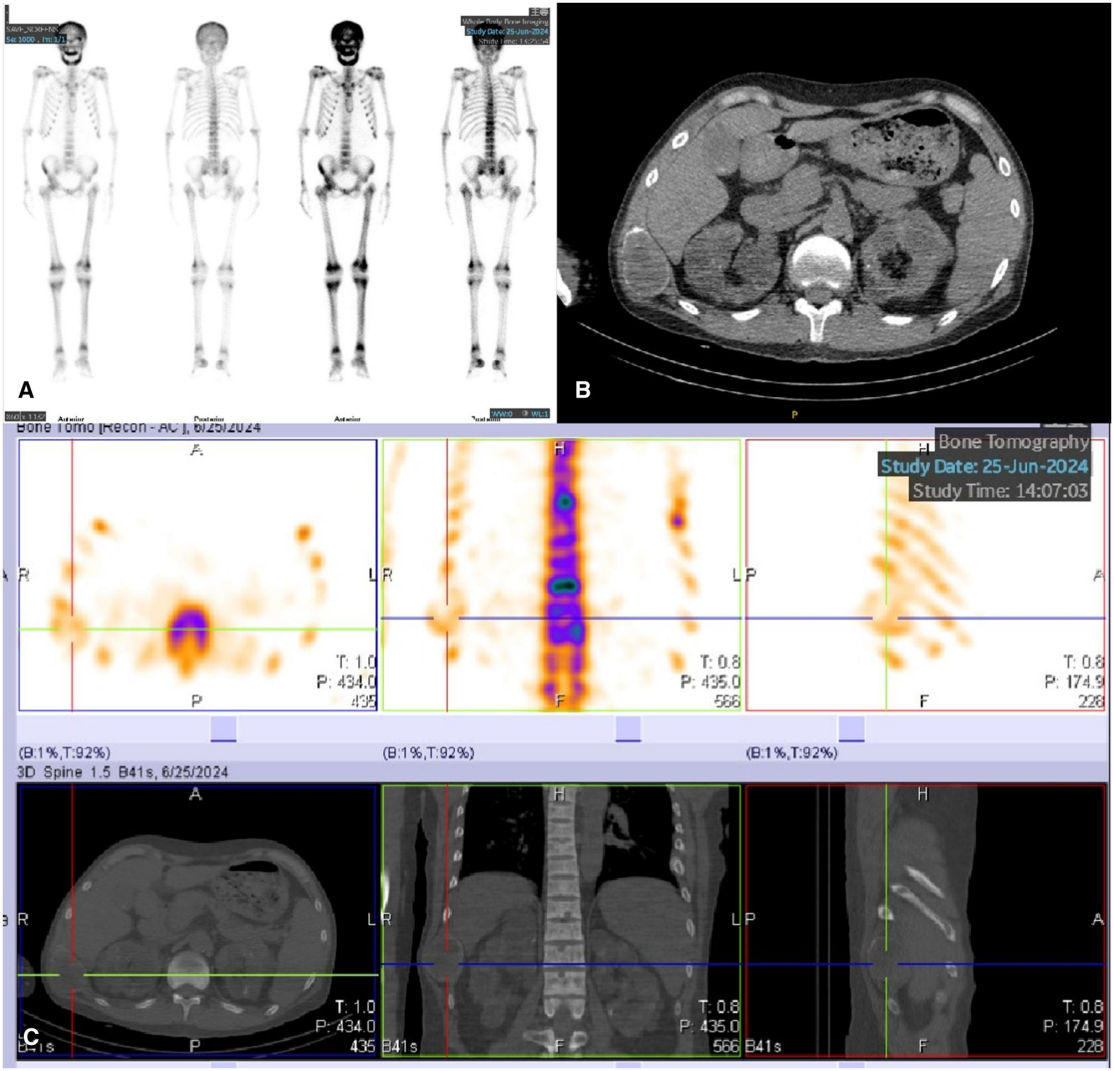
(A) The whole-body bone scan demonstrates diffuse tracer uptake in the skull, with abnormally increased radiotracer accumulation observable in the clavicles, ribs, sternum, scapulae, long bones of the extremities, pelvic bones, and vertebral column. These findings are suggestive of metabolic bone disease, most likely secondary to hyperparathyroid dysfunction. (B, C) SPECT/CT tomographic imaging demonstrates abnormally increased uptake of bone imaging agents. A hyperdense, well-demarcated, expansile osteolytic lesion is observable in the right 10th rib with thinning of the surrounding cortical bone. The left 6th and 7th ribs, as well as the right 11th rib, show irregular morphology with partial expansion, reduced bone density, and evidence of callus formation in some regions. These findings suggest a brown tumour in the right 10th rib and pathological fractures in the left 6th and 7th ribs and right 11th rib.
